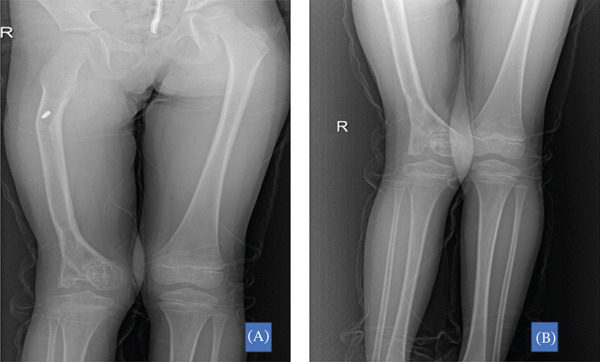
Eighteen‐month follow‐up radiograph showing alignment of (A) both lower limbs showing (B) both femurs showing both knees.
